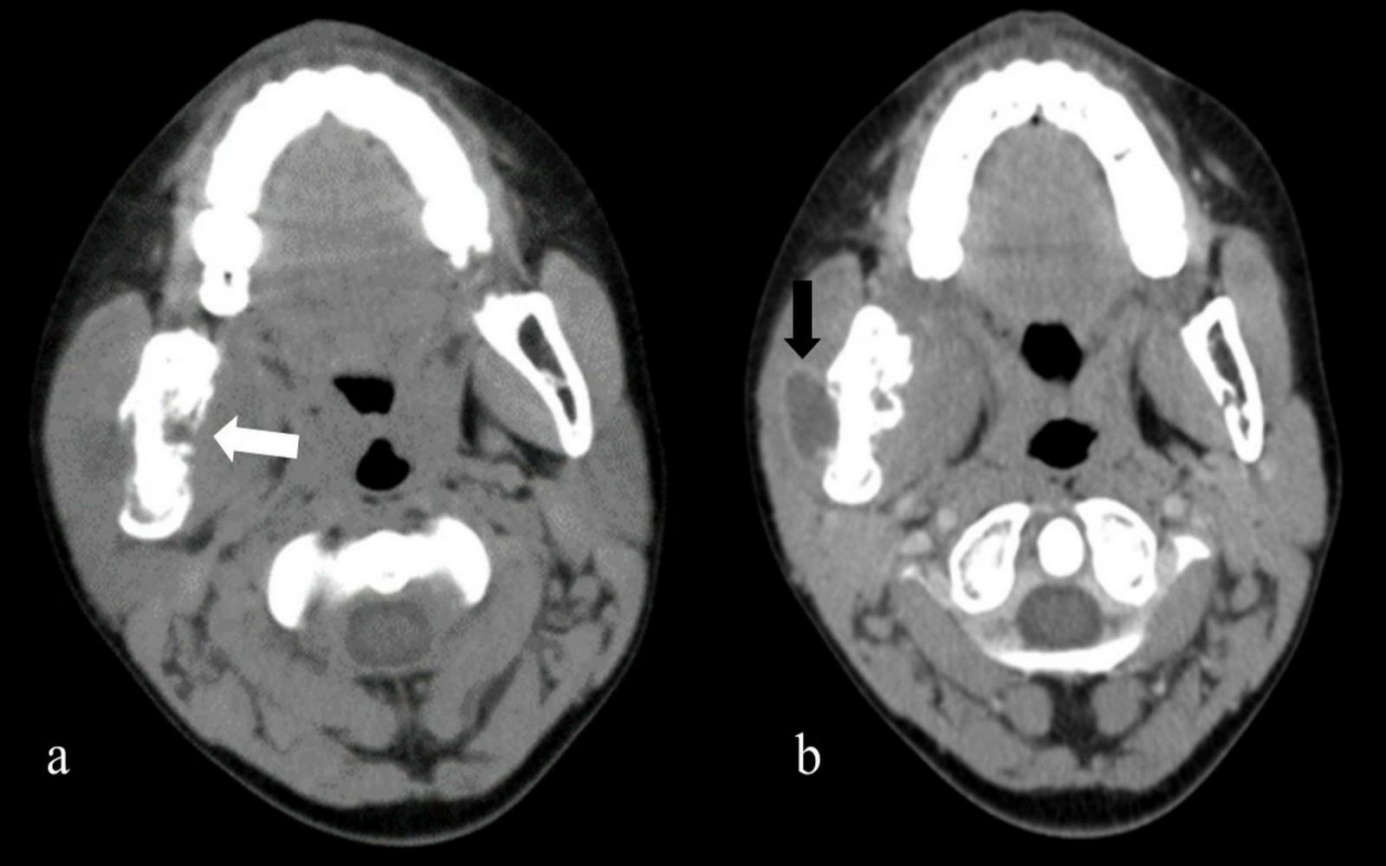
a) Contrast-enhanced computed tomography head axial view showing irregularly thickened cortex with loss of corticomedullary junction with multiple loosened areas showing a breach of the cortex and periosteal reaction (white arrow) with adjacent soft tissue component. b) Showing central hypoattenuation areas representing central necrosis (black arrow) with periosteal reaction
